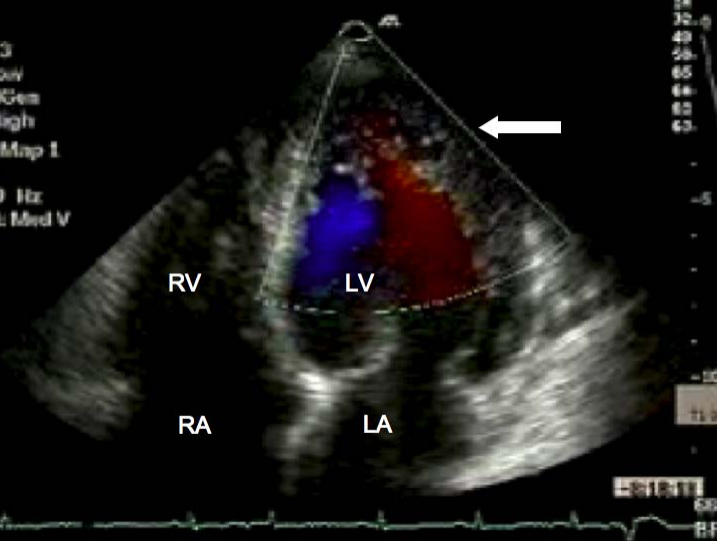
Transthoracic echocardiography. Doppler colour flow mapping suggesting blood flow present between the ventricular trabeculations (arrow). RA, right atrium; LA, left atrium; LV, left ventricle; RV, right ventricle.
